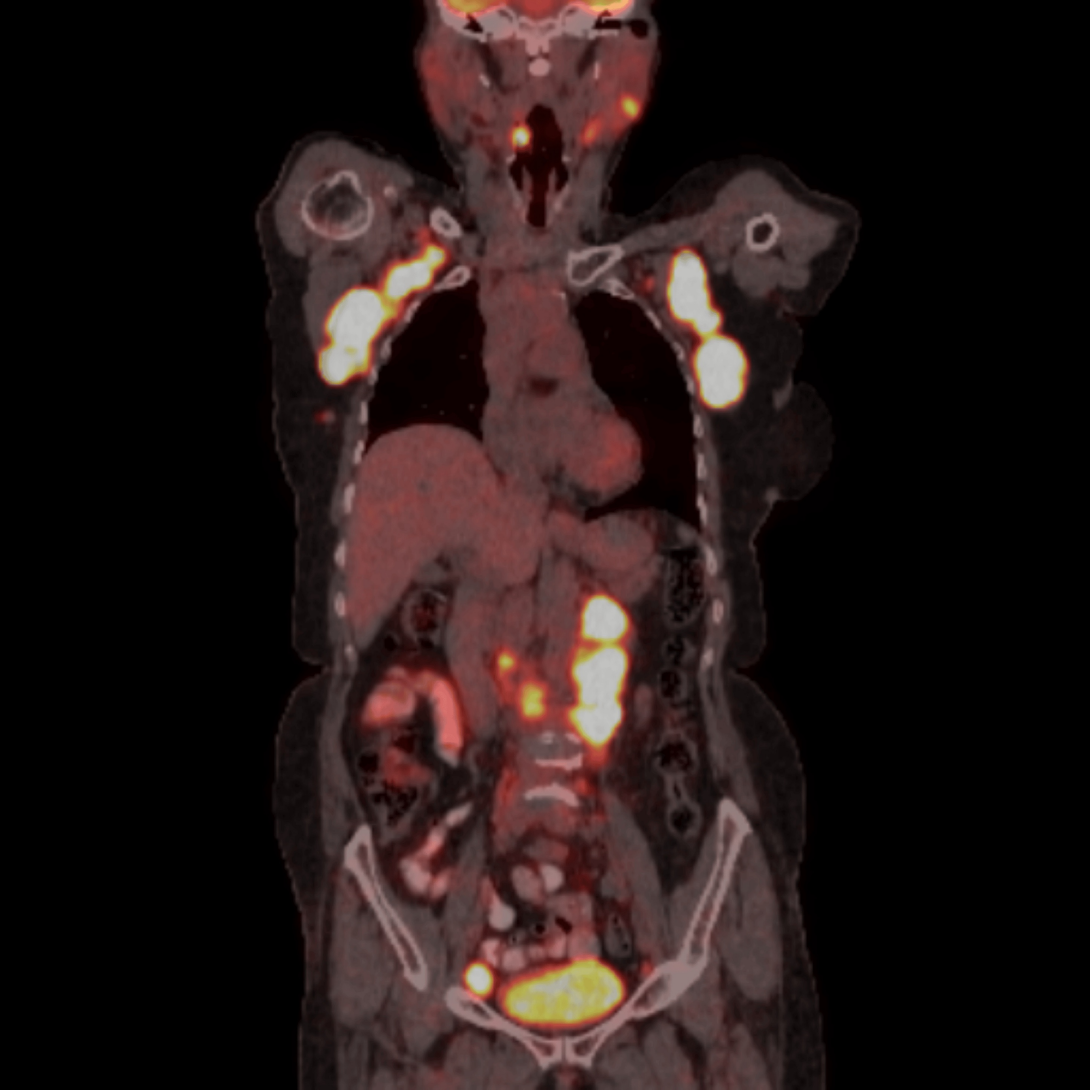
Coronal hybrid fused fluorodeoxyglucose (FDG) positron emission tomography-computed tomography (PET-CT) images revealing multiple FDG-avid supra and infra-diaphragmatic lymph nodes, suggesting involvement by high-grade non-Hodgkin lymphoma.
